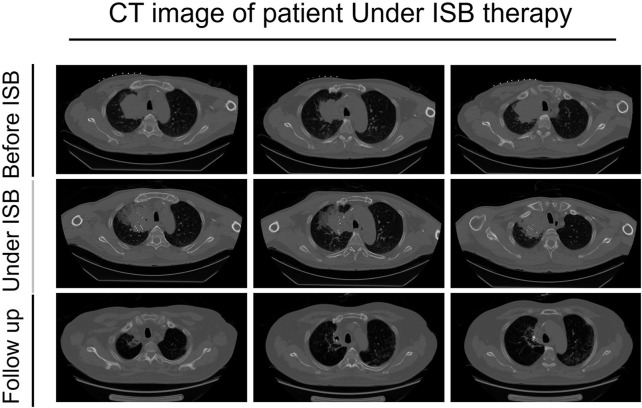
CT images of the patient before using ISB treatment, under the treatment, and follow-up after the treatment.
